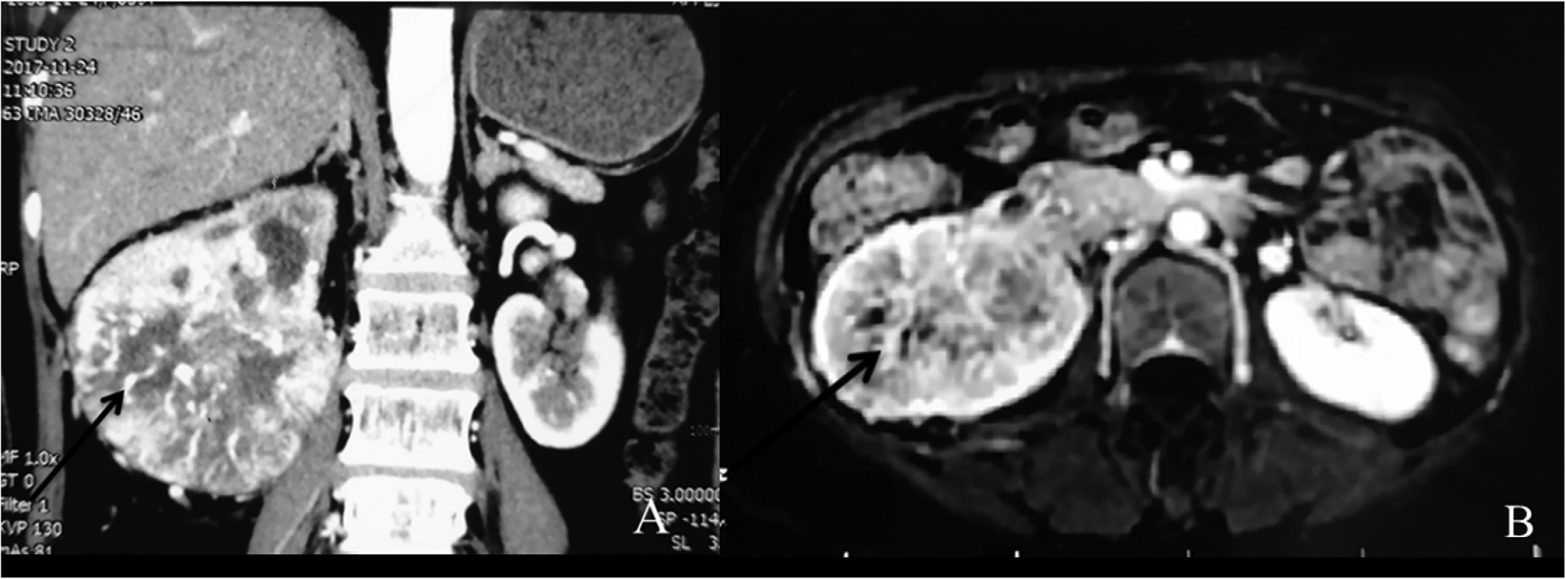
MRI findings and an expelled worm. Axial and coronal MRI revealed that the right kidney was enlarged and severely damaged (2A and 2B).
